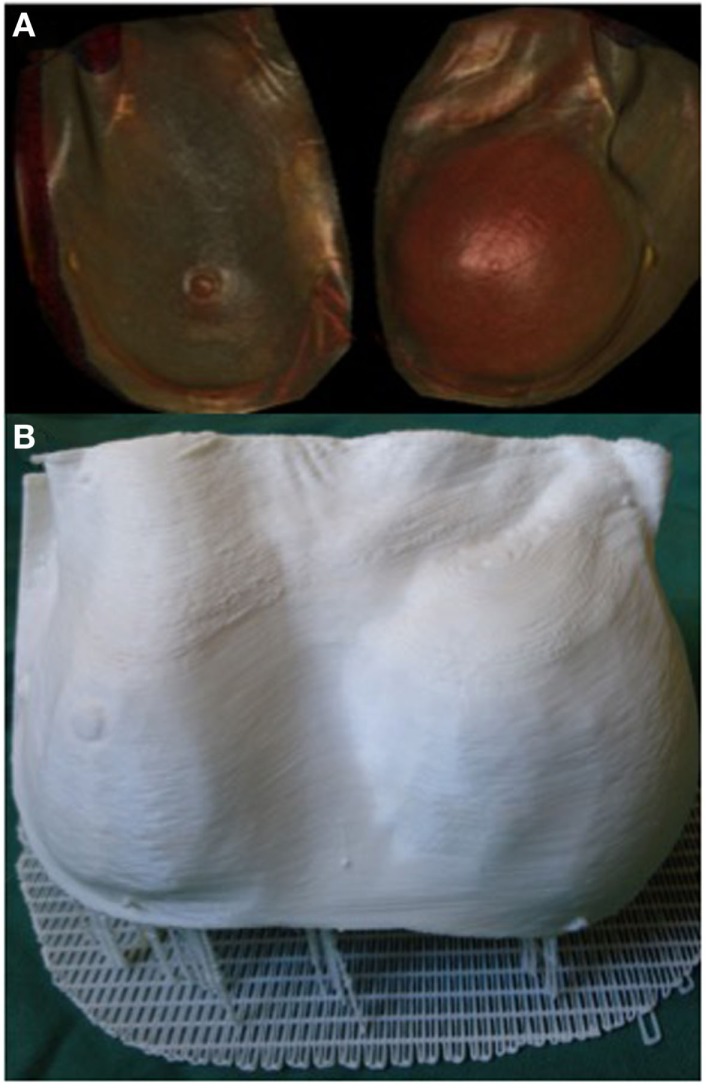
3D reconstructed CT images of a patient with breast asymmetry post-mastectomy (A) and the 3D printed breast model of the same patient (B). Reproduced with permission from *Breast Cancer Research and Treatment* (108).
